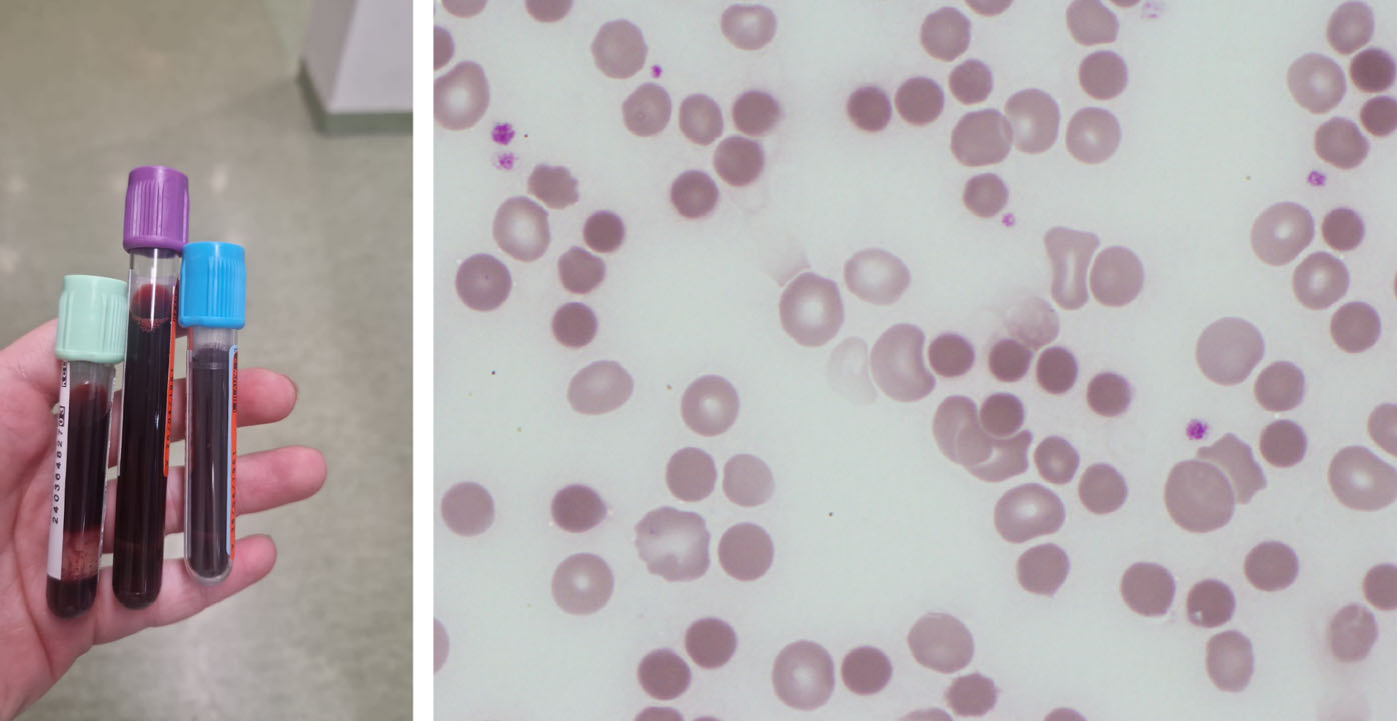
A 70‐year‐old woman with a history of successfully resected pancreatic cancer 10 years ago presented with dyspnea and fever. Laboratory tests showed leukocytosis (white cell count 20.8 × 10^9^/L) and elevated C‐reactive protein (87 mg/L) with normal hemoglobin concentration (Hb) (137 g/L) and platelet count (242 × 10^9^/L). Computed tomography identified a hepatic abscess. The abscess was drained and ceftriaxone‐metronidazole was administered intravenously. Sixteen hours after presentation, the patient's condition deteriorated, with Hb dropping to 50 g/L while the platelet count remained normal. Macroscopically the blood sample appeared dark (left image), and no red blood cells could be separated upon centrifugation. A blood film showed spherocytosis and dehemoglobinized ghost cells (right image, May–Grünwald–Giemsa ×100 objective), indicating acute intravascular hemolysis. Despite early drainage plus antibiotic treatment, and admission to the intensive care unit, the patient died 21 h after initial presentation. Blood and abscess cultures grew *Clostridium perfringens* (metronidazole susceptible).
